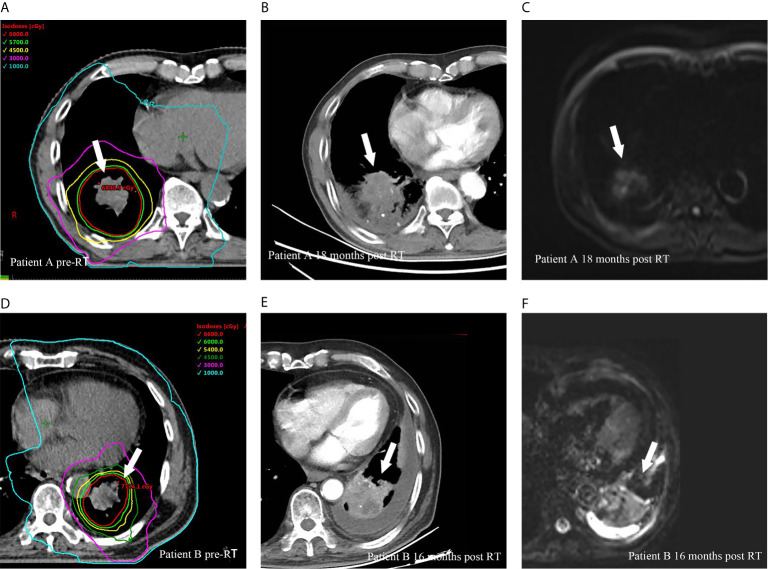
Representative cases of local recurrences within SIB region for two patients after HypoRT treatment. Planning computed tomography fused with isodose levels before RT courses for patient A (A), diagnostic computed tomography (B) and magnetic resonance diffusion weighted imaging (C) showed a local recurrence (arrows) 18 months after RT. (D–F) presented the planning imaging, the local recurrence in diagnostic computed tomography and magnetic resonance diffusion weighted imaging 16 months after RT for patient B.
